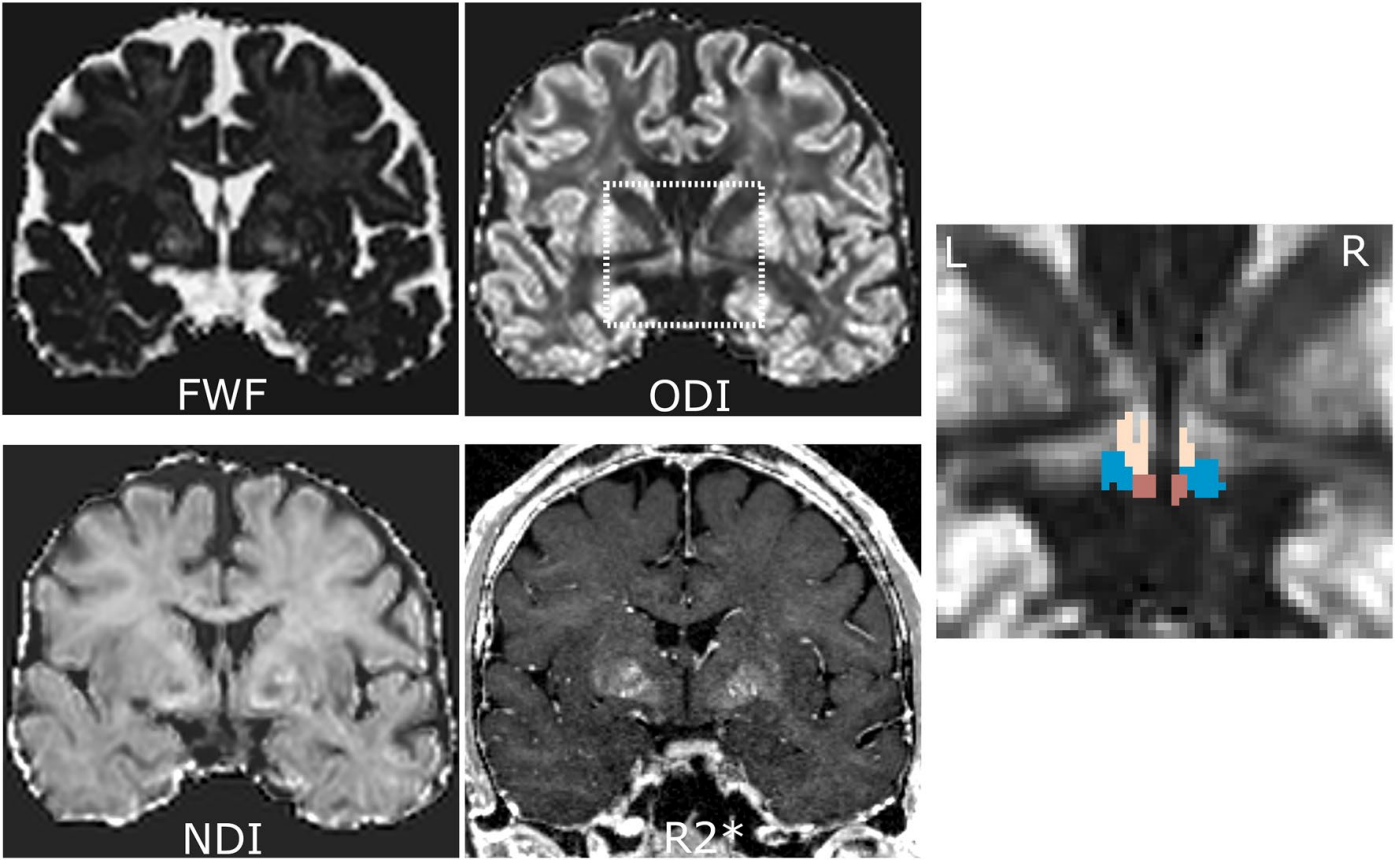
Exemplary dataset in native space displaying the four used MRI parameters in coronal view (FWF: free water fraction, ODI: orientation dispersion index corrected for FWF, NDI: neurite density index corrected for FWF, R2*: effective transverse relaxation rate), as well as the ODI image zoomed in on the hypothalamus area and overlaid with the hypothalamus masks after correction for confounding white matter (beige: superior, blue: intermediate, brown: posterior subunits). The inferior subunit is not visible on this slice.
